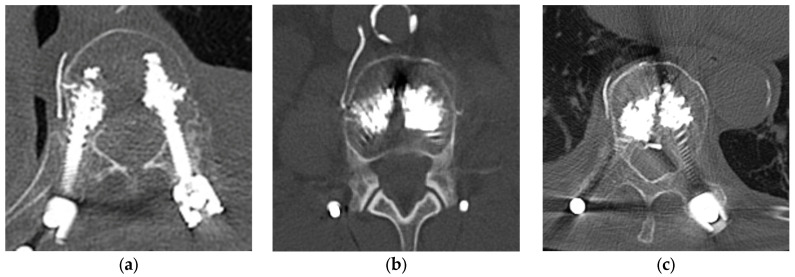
Representative CT images of the cement leakage classification. (a) Leakage into the segmental vein (Type S); (b) Leakage into the inferior vena cava (Type S); (c) Leakage into the segmental vein and the spinal canal (Type S + B).
